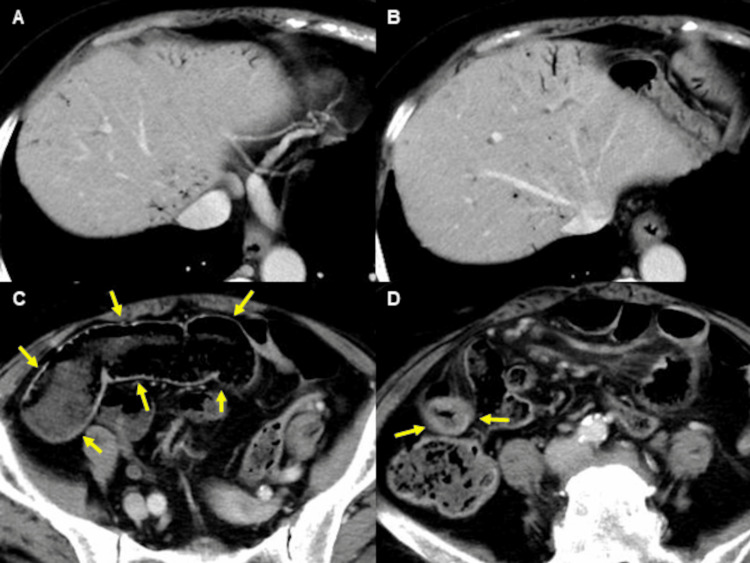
Emergency CT shows hepatic portal venous gas (HPVG) (panels A and B) and ileal dilatation (panel C, arrows) involving focal stenosis of the ileum (panel D, arrows).
